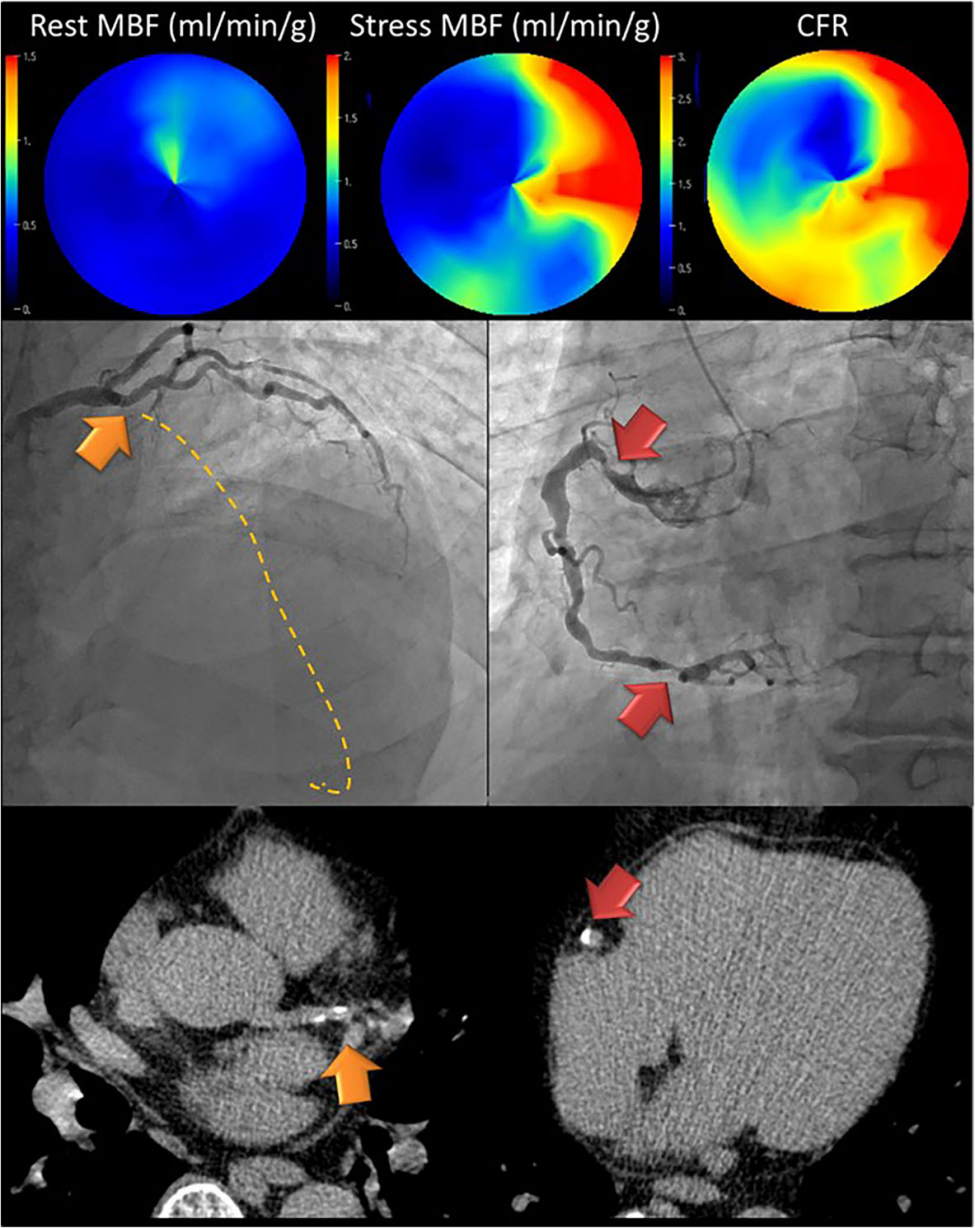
Representative CAD case: A man in his 60s who had significant stenosis in the LAD (#6 100%; *orange arrow*) and RCA (#1 70%, #3 90%; *red arrows*). ^15^O-water PET/CT demonstrated the decrease of stress MBF and CFR in the LAD and RCA territories. The estimated whole CFR was 2.19. The CFRs in the LAD, LCX, and RCA territory were 1.66, 3.11, and 2.01, respectively. Calcifications were seen on the LAD and RCA (Agatston score = 97)
